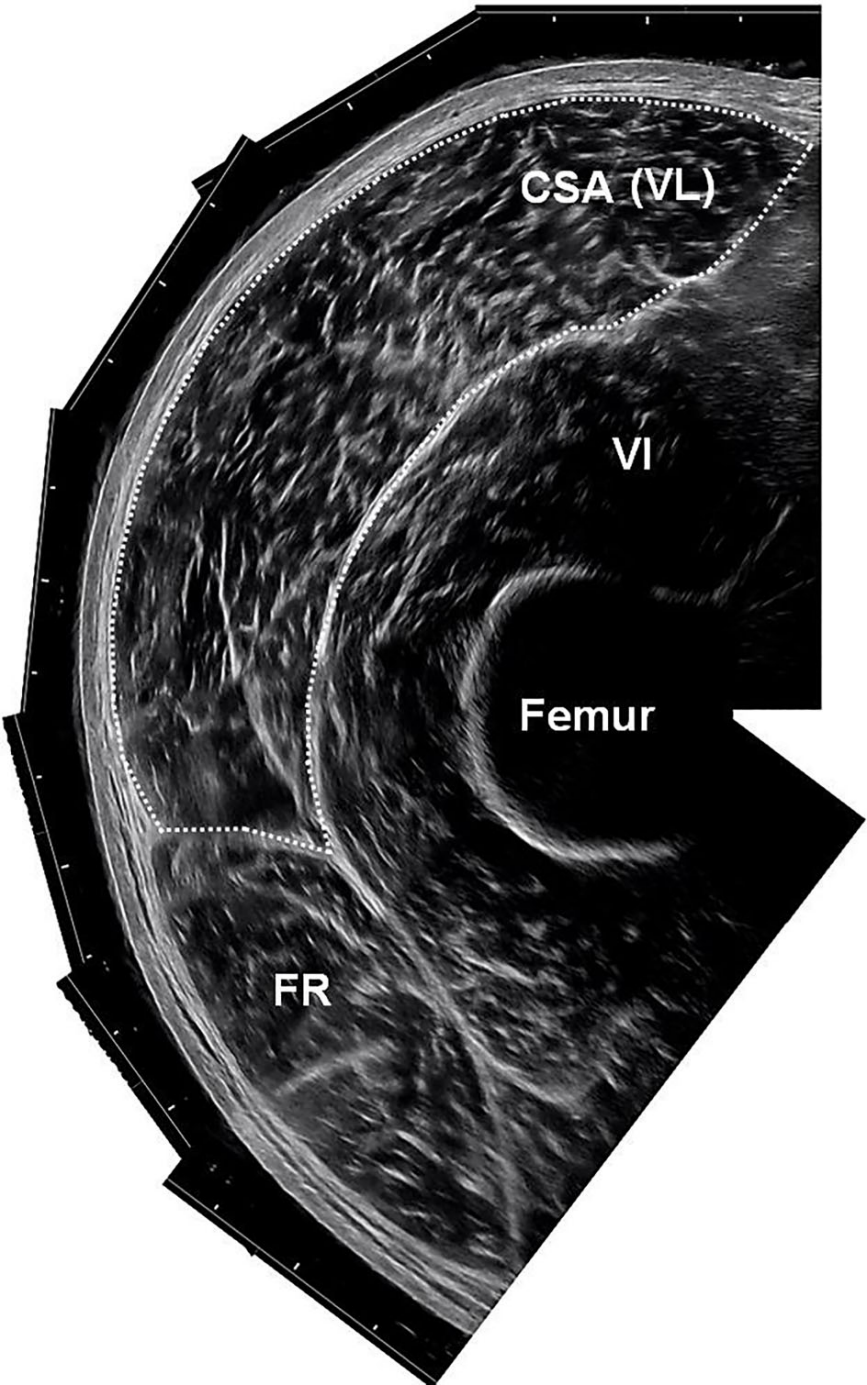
Reconstitution of the ultrasound image of the vastus lateralis (VL) and demarcation of the cross-sectional area (CSA). VI: vastus intermedius; FR: rectus femoris.
